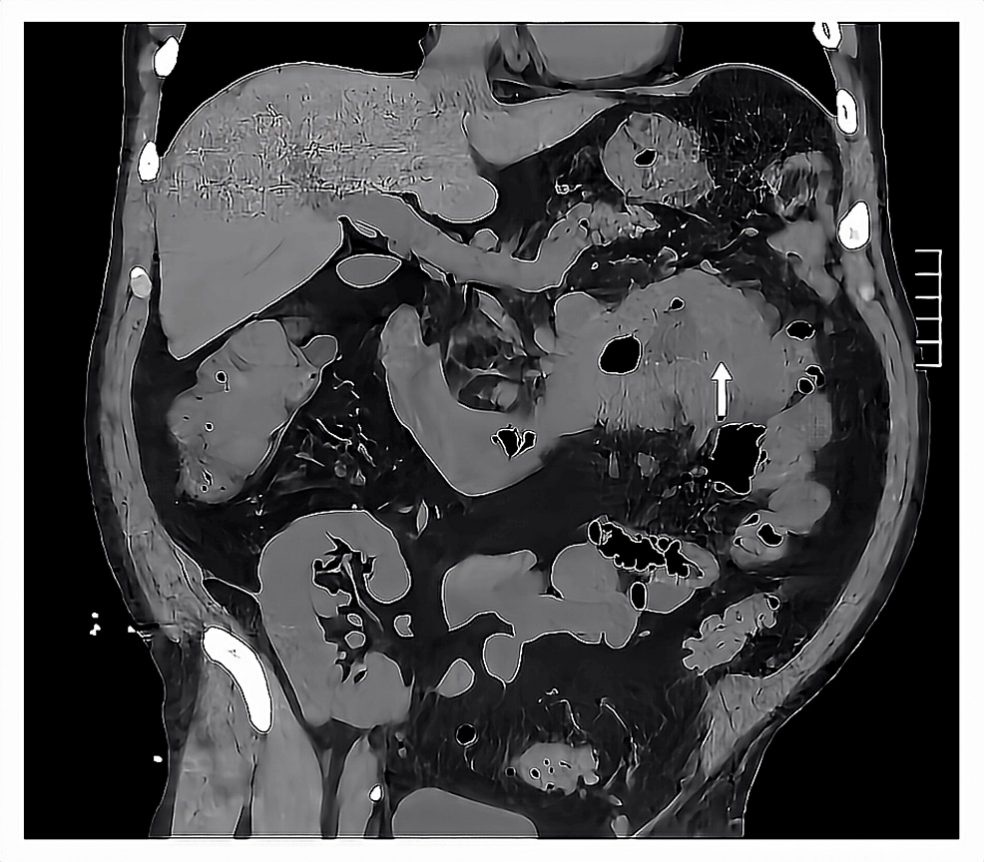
CT of the abdomen and pelvis showing a large mass in the duodenum (white arrow).
